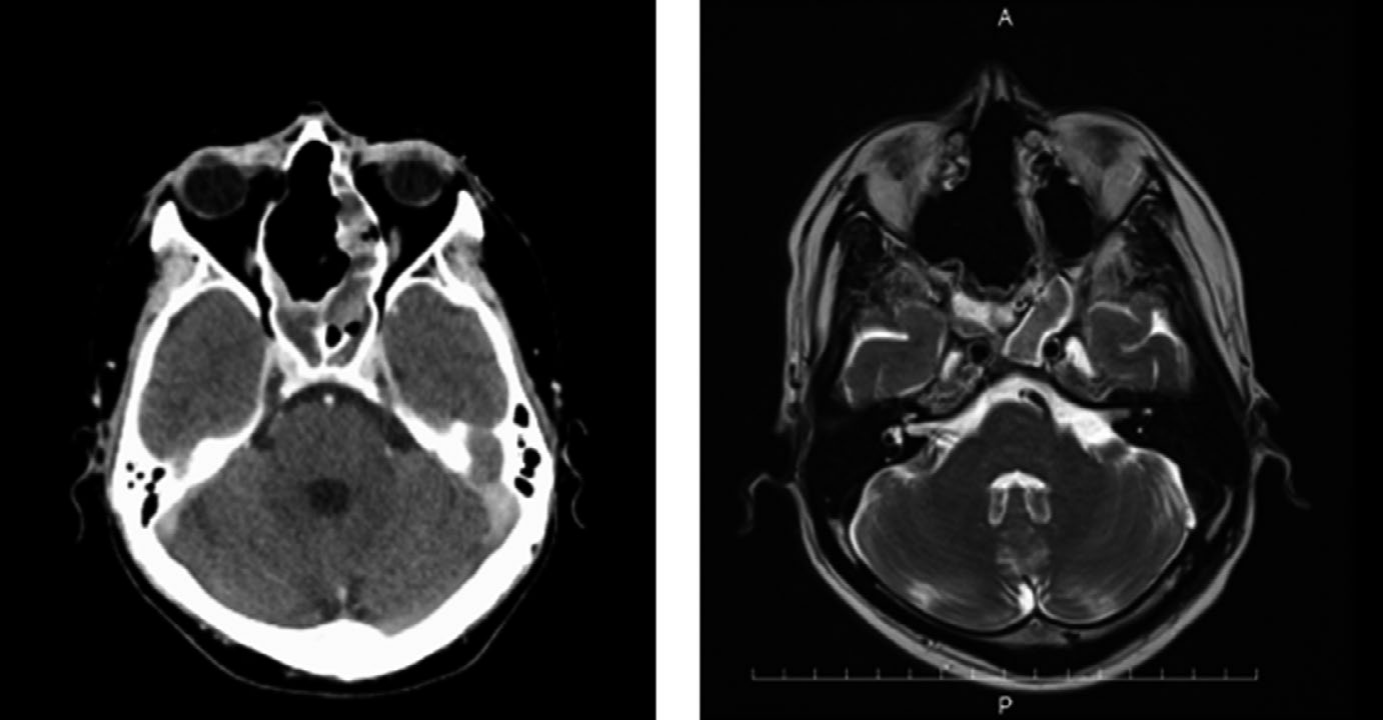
Computed tomography and magnetic resonance images showing complete tumor resection with preserved anterior ethmoid bone
